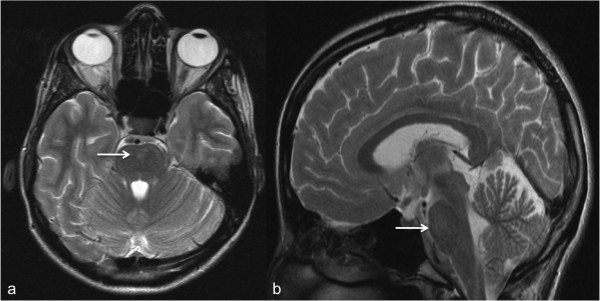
Brainstem abnormalities in a 28-year-old patient. (a) Axial and (b) sagittal T2w- TSE MR- images of the brain show hyperintense signal alterations in the pons (arrows). T2w, T2-weighted; TSE, turbo spin-echo; MR, magnetic resonance.
